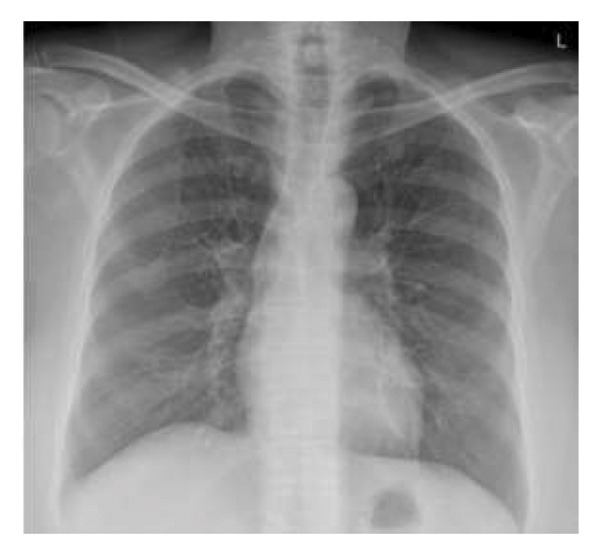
Normal chest radiograph.
